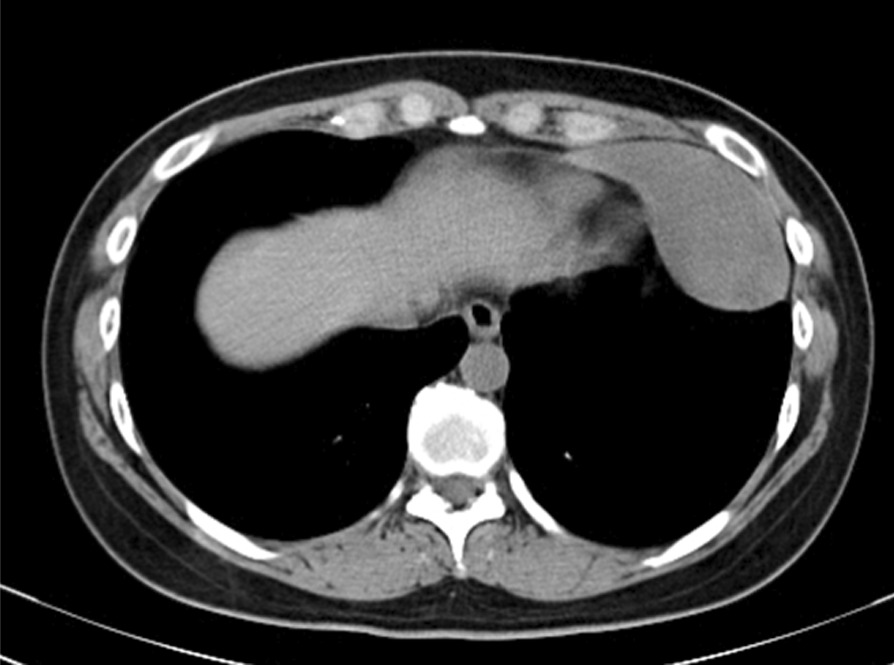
Chest computed tomography image of the tumor. The tumor was located on the pericardium and did not seem to invade the adjacent organs. The tumor had a pedicle on the pericardium
